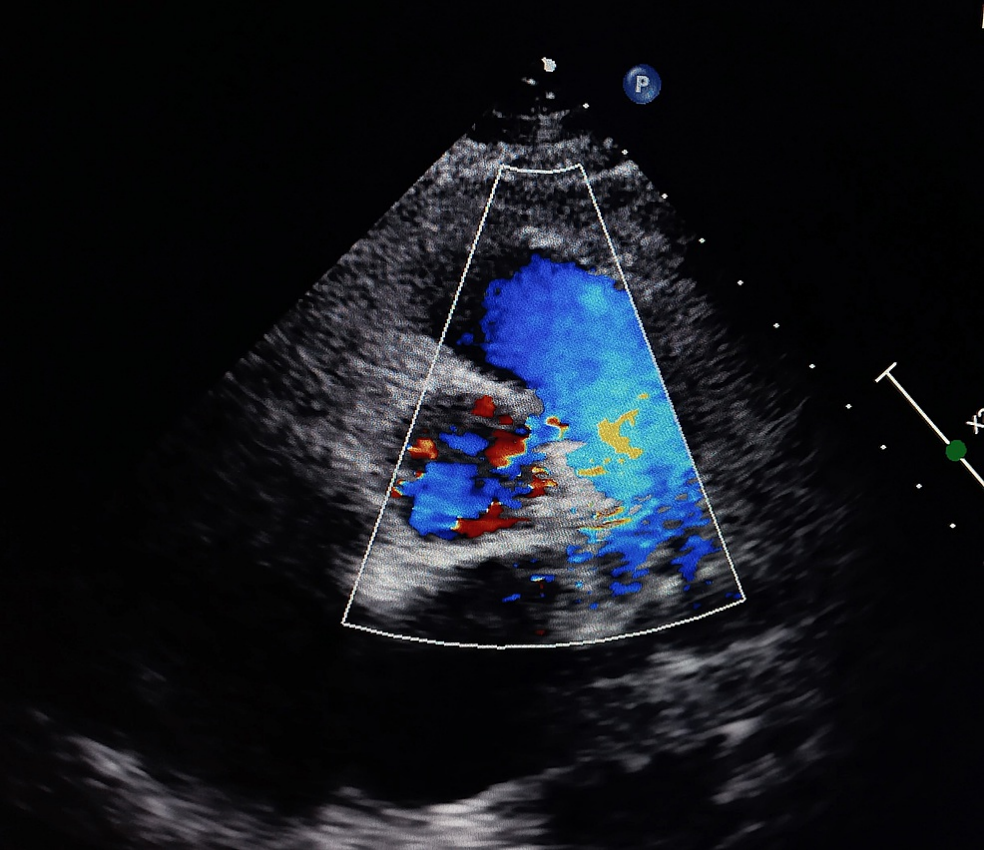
Color Doppler echocardiographic view showing flow across the aortopulmonary septal defect
